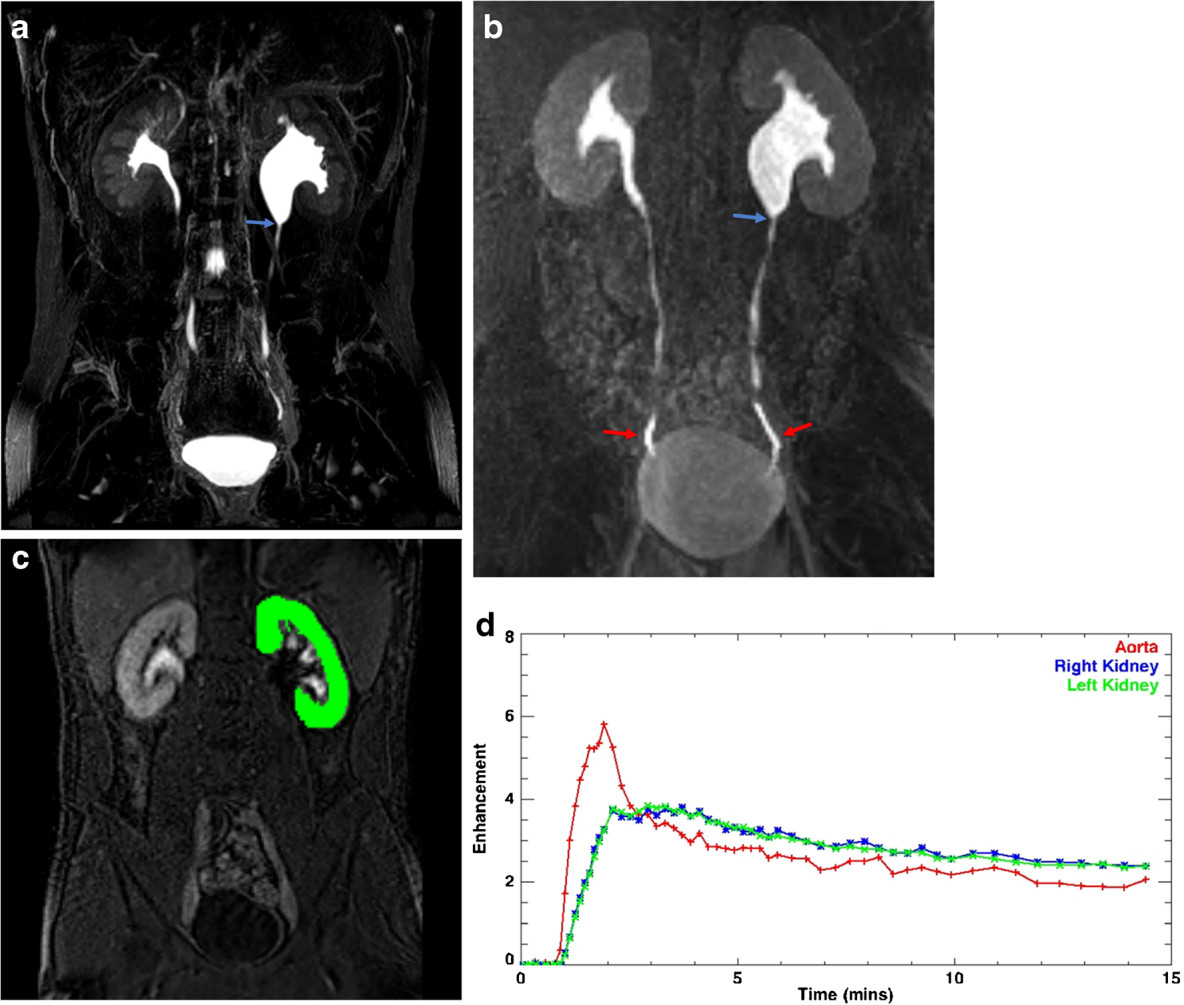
Seventeen-year-old with left hydronephrosis and cyclic vomiting who has previously undergone left pyeloplasty. a Maximum intensity projection (MIP) reconstruction of 3D T2-weighted fast spin-echo image showing abrupt narrowing of the left renal pelvis at the level of the ureteropelvic junction (arrow). b MIP reconstruction of a post-contrast excretory image shows symmetric renal enhancement and excretion of contrast. Contrast readily flows past the narrowed, yet patent UPJ (blue arrow) as evidenced by the presence of contrast within both distal ureters (red arrows). On dynamic imaging, there was symmetric passage of contrast material through the kidneys and into the renal collecting systems and ureters suggesting no clinically relevant obstruction. c Example of region of interest overlying the left kidney performed during segmentation. d Time-vs.-signal intensity curves from the kidneys and abdominal aorta are obtained from dynamic post-contrast MR urograms post-processing; in this case, the curves demonstrate symmetric renal uptake and excretion confirming lack of obstruction in the left kidney
